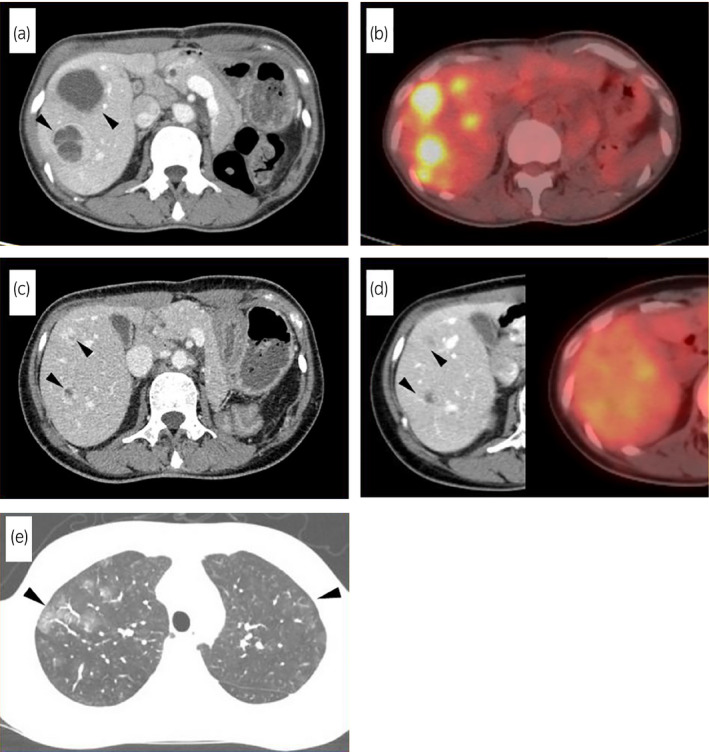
(a) At 4 months after starting EDP‐M therapy, contrast‐enhanced CT revealed that the multiple liver metastases had increased in size (black arrowheads). (b) At the same time, PET‐CT showed that the multiple liver metastases had grown larger, and the SUVmax was 8.8. (c) At 7 months after starting GC‐M, CT revealed that the multiple liver metastases had shrunk in size (black arrowheads). (d) CT and PET‐CT showed a partial response at over 12 months after starting GC‐M (black arrowheads). (e) CT showed granular shadows in the bilateral lung (black arrowheads).
